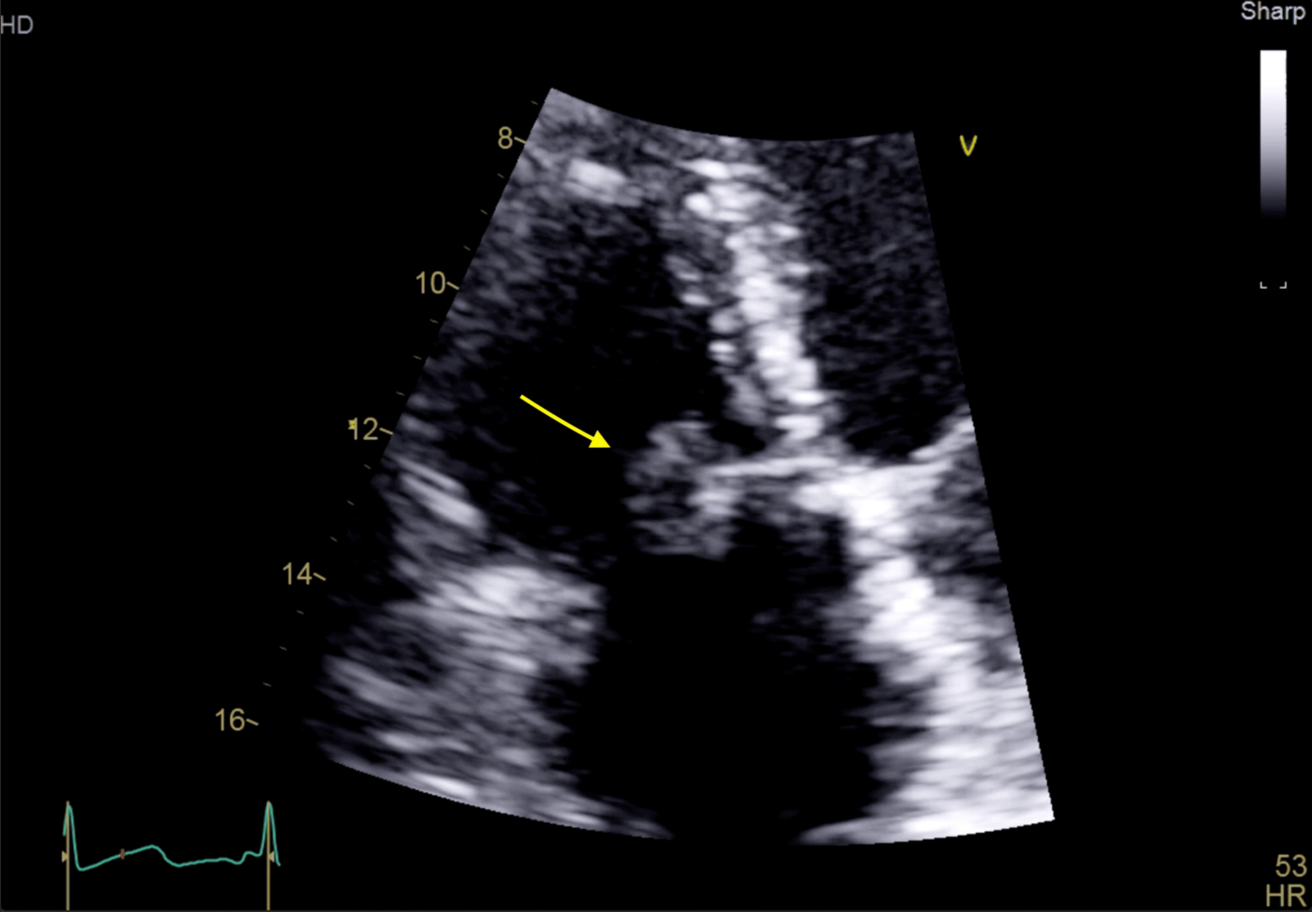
Transthoracic echocardiogram (apical four-chamber view) demonstrating a large mobile mass on the tricuspid valve. The arrow annotation highlights high-density mass on the anterior leaflet of the TV. TV: tricuspid valve
